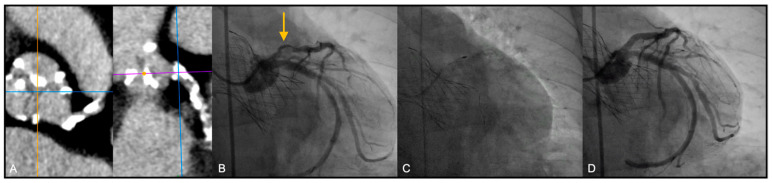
Rotational atherectomy (RA) following Medtronic CoreValve deployment by Trans-Femoral approach. (A) Pre-TAVI CT views showing severe calcification of LAD; (B) Pre RA angiographic evaluation of the lesion: proximal LAD presented severe calcified lesion (arrow); (C) Multiple burr (RotaPro 1.25 mm) advancements; (D) Final result, after stent deployment and post dilation.
